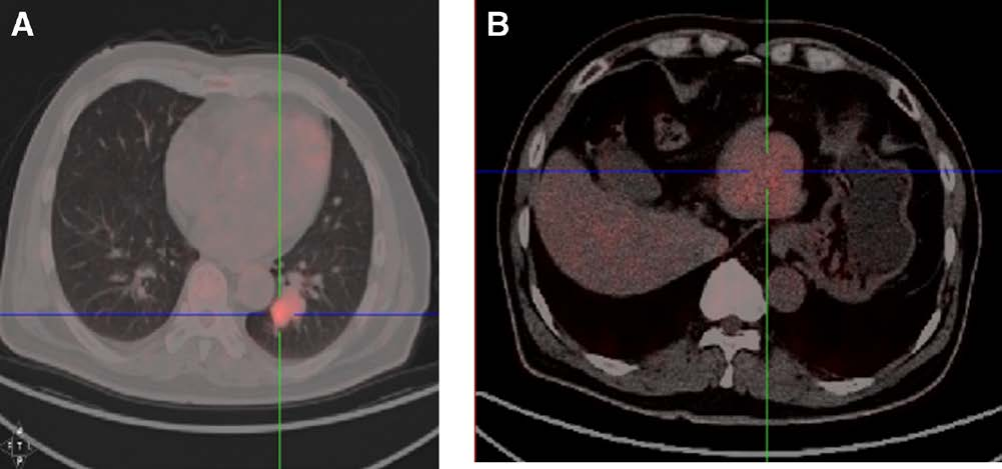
Baseline 18F-FDG PET/CT revealed an intense FDG uptake (A) in the pulmonary lesion (SUV_max_ 8.2; left) and (B) in the left-lobe hepatic mass (SUV_max_ 5.2; right), supporting dual primary malignancies. CT = computed tomography, FDG = fluorodeoxyglucose, PET = positron emission tomography, SUV = standard uptake value.
